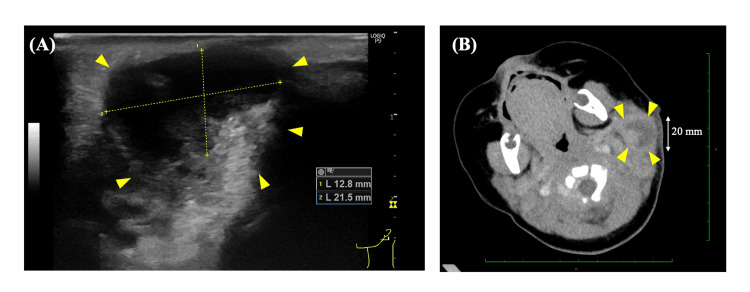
Imaging Studies of Suppurative Lymphadenitis Panel A: Cervical ultrasonography demonstrating an enlarged lymph node in the left preauricular region measuring 12.8 mm × 21.5 mm. The lymph node shows hypoechoic areas (yellow arrowheads) suggestive of liquefaction and abscess formation. Yellow measurement calipers indicate the two dimensions of the largest lymph node. Panel B: Contrast-enhanced computed tomography (CT) of the neck in axial view, showing multiple enlarged lymph nodes in the left parotid region with central low-density areas (yellow arrowheads) consistent with abscess formation. The scale bar indicates 20 mm. No airway compromise or extension into deeper neck spaces is evident. These images were obtained at Juntendo University Shizuoka Hospital as part of routine clinical care. Written informed consent for publication of these anonymized images was obtained from the patient's parents. All identifiable information has been removed to protect patient privacy. CT, computed tomography.
